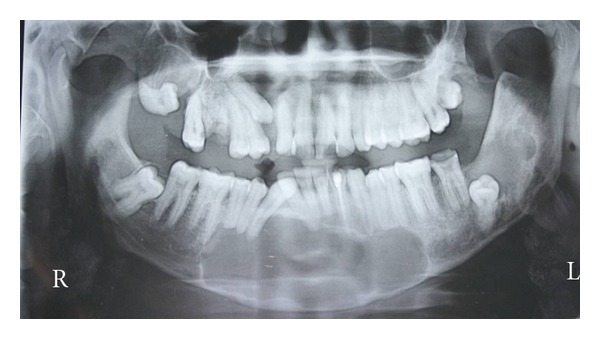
Orthopantomograph shows multiple radiolucencies associated with mandibular anterior region, maxillary right and left as well as mandibular left impacted third molar teeth.
